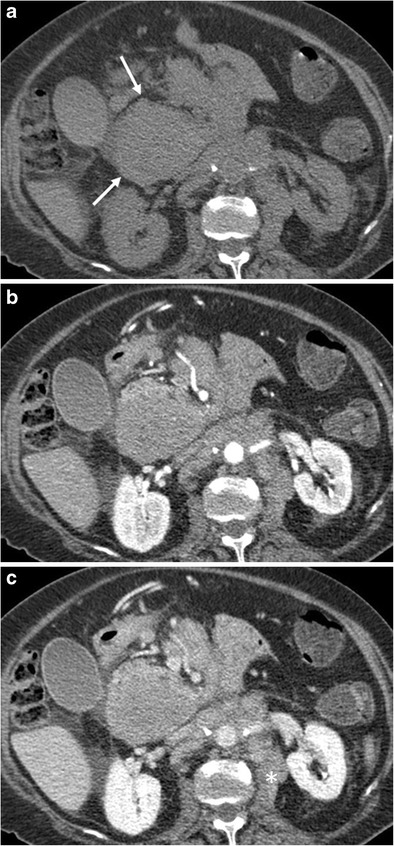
A 79-year-old female patient with primary pancreatic follicular lymphoma (Ki 67 score < 25%). a Unenhanced CT scan depicts a hypodense mass in the pancreatic head (arrows). b, c CT images after contrast medium administration in the arterial-pancreatic (b) and portal-venous phase (c): progressive enhancement of the neoplasm is visible. Enlarged retroperitoneal lymph nodes with ill-defined margins are depicted (*)
